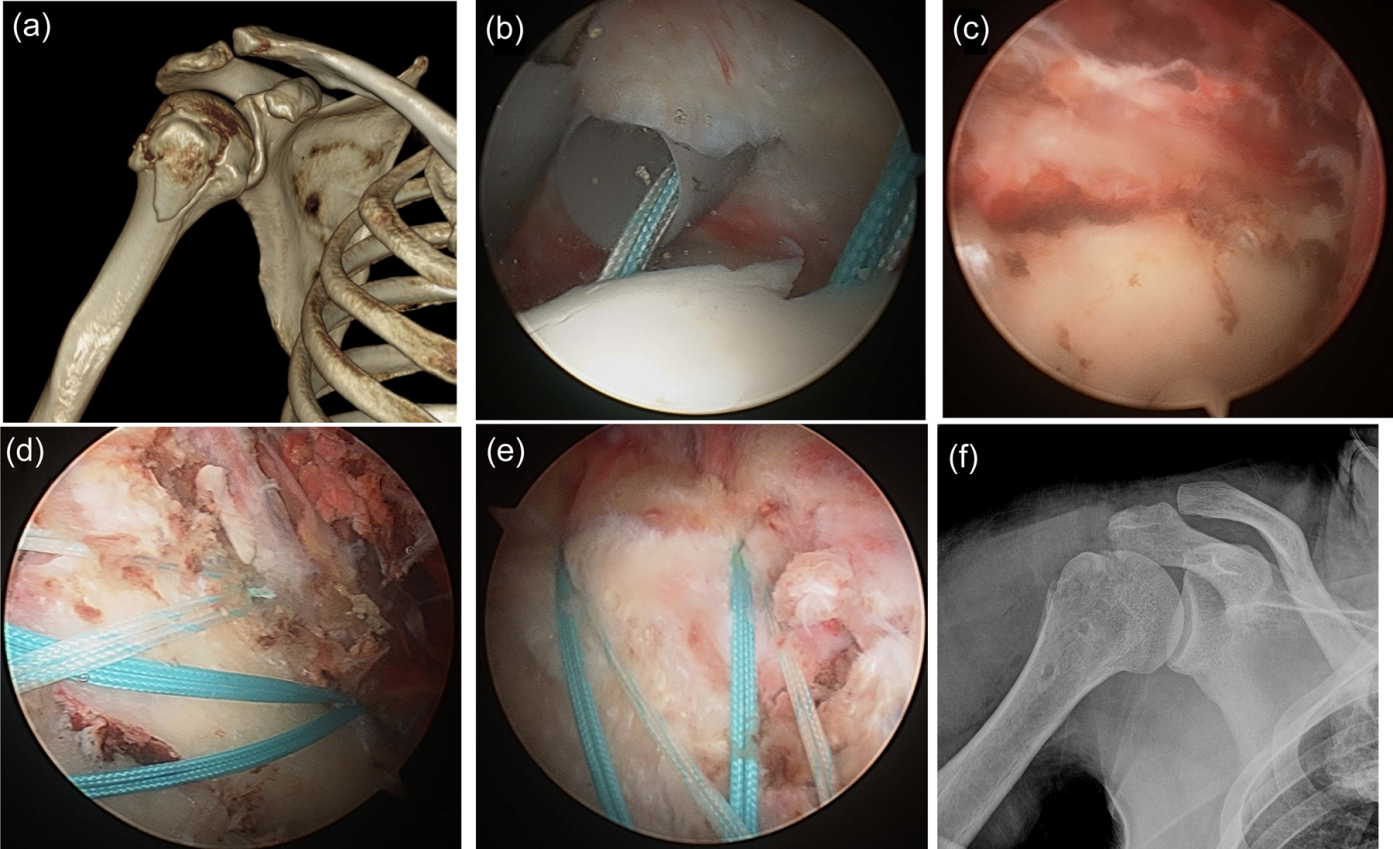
Arthroscopic reduction and double‐row suture bridge fixation for large greater tuberosity fractures of the proximal humerus. (a) Three‐dimensional computed tomography confirming a large greater tuberosity fracture. (b) Insertion of two double‐loaded suture anchors into the medial side of the fracture fragment through the trans‐cuff portal. (c) Verification of the fracture site in the subacromial space. (d) Achieving double‐row suture bridge fixation by placing two lateral anchors into just posterior to the bicipital groove. (e) Demonstration of knotless fixation on the medial side of the fracture site. (f) Immediate postoperative radiograph showing a well‐reduced fracture site.
